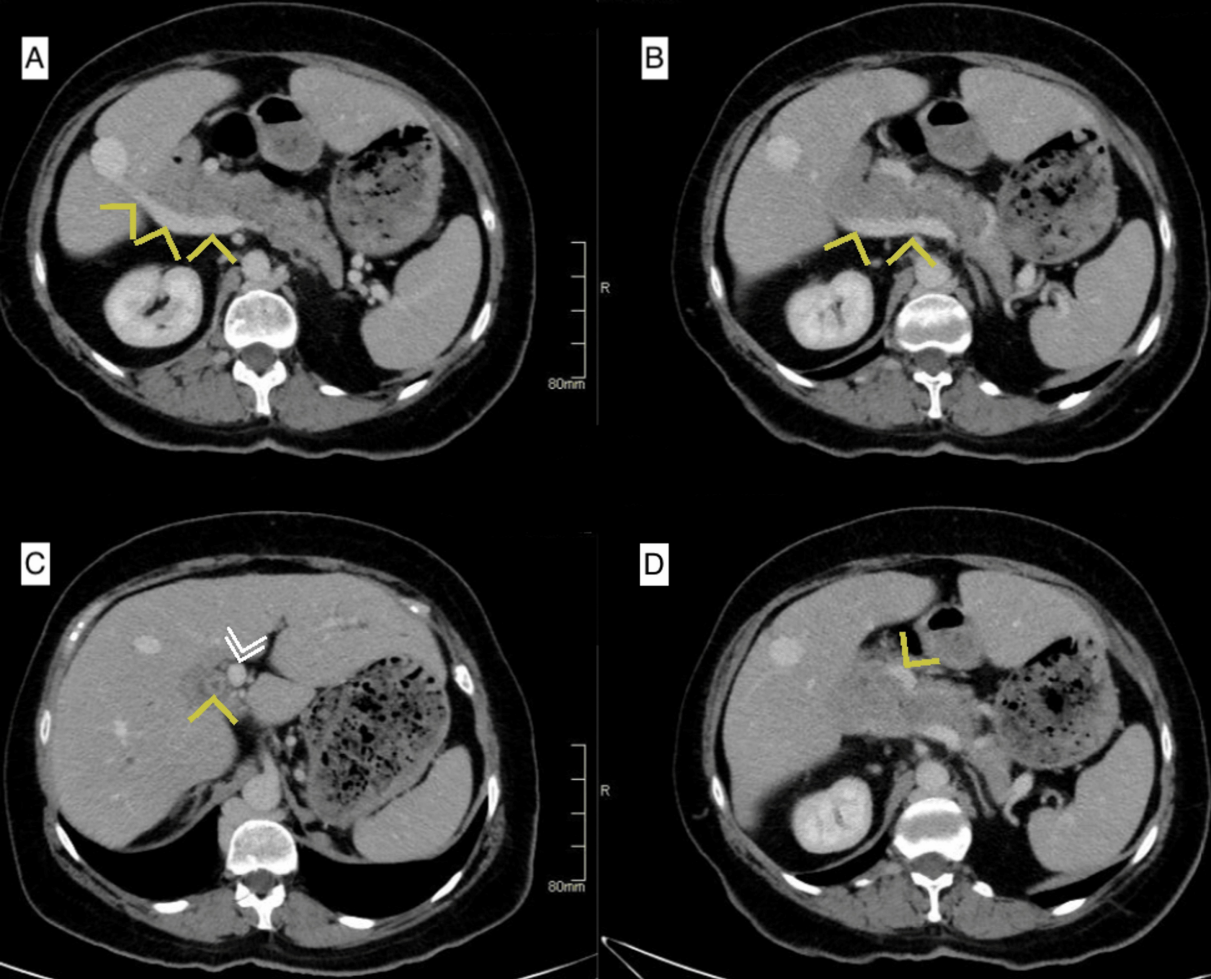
Computed tomography images showing the aberrant anatomy of the second portal vein (yellow arrows) traveling behind the pancreas and entering the liver near segment VI (A, B), a stone in the common bile duct (yellow arrow) next to the original portal vein (white double arrows) at the level of the porta hepatis (C), and the original portal vein (yellow arrow) coursing anterior to the pancreas (D).
